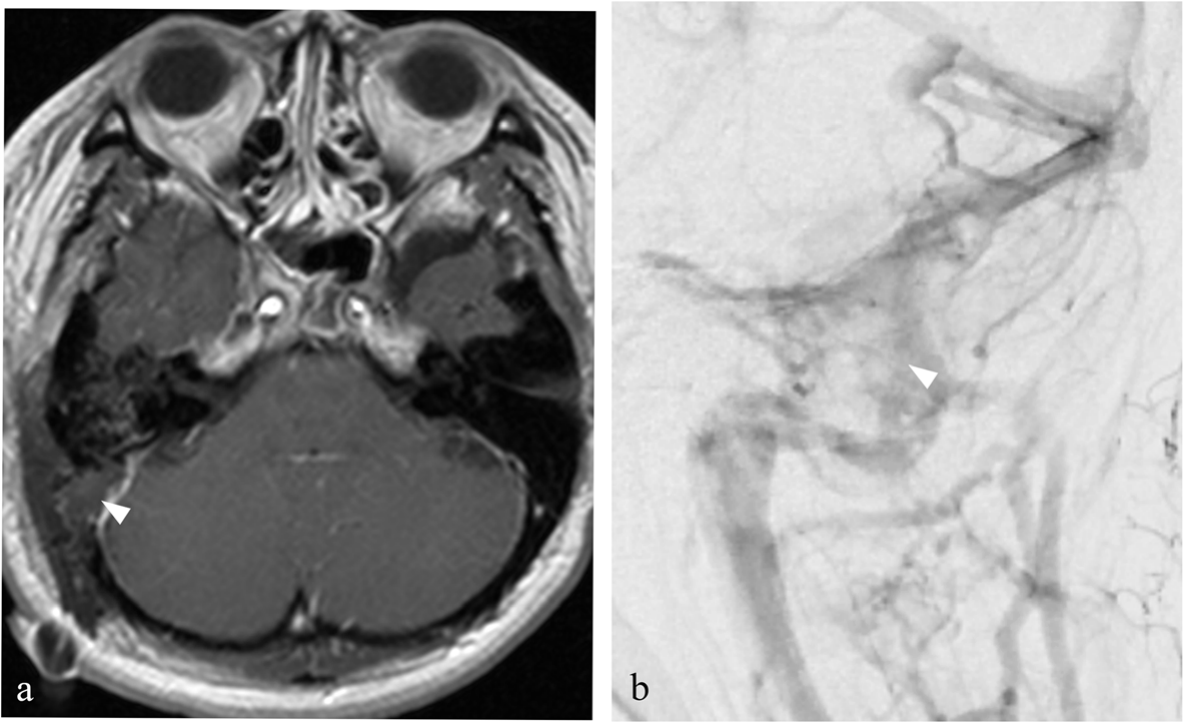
a Postoperative gadolinium-enhanced T1-weighted magnetic resonance images showing removal of the tumor (*arrowhead*). b Postoperative cerebral angiography showed patency of the left transverse and sigmoid sinuses showing removal of the tumor (*arrowhead*)
